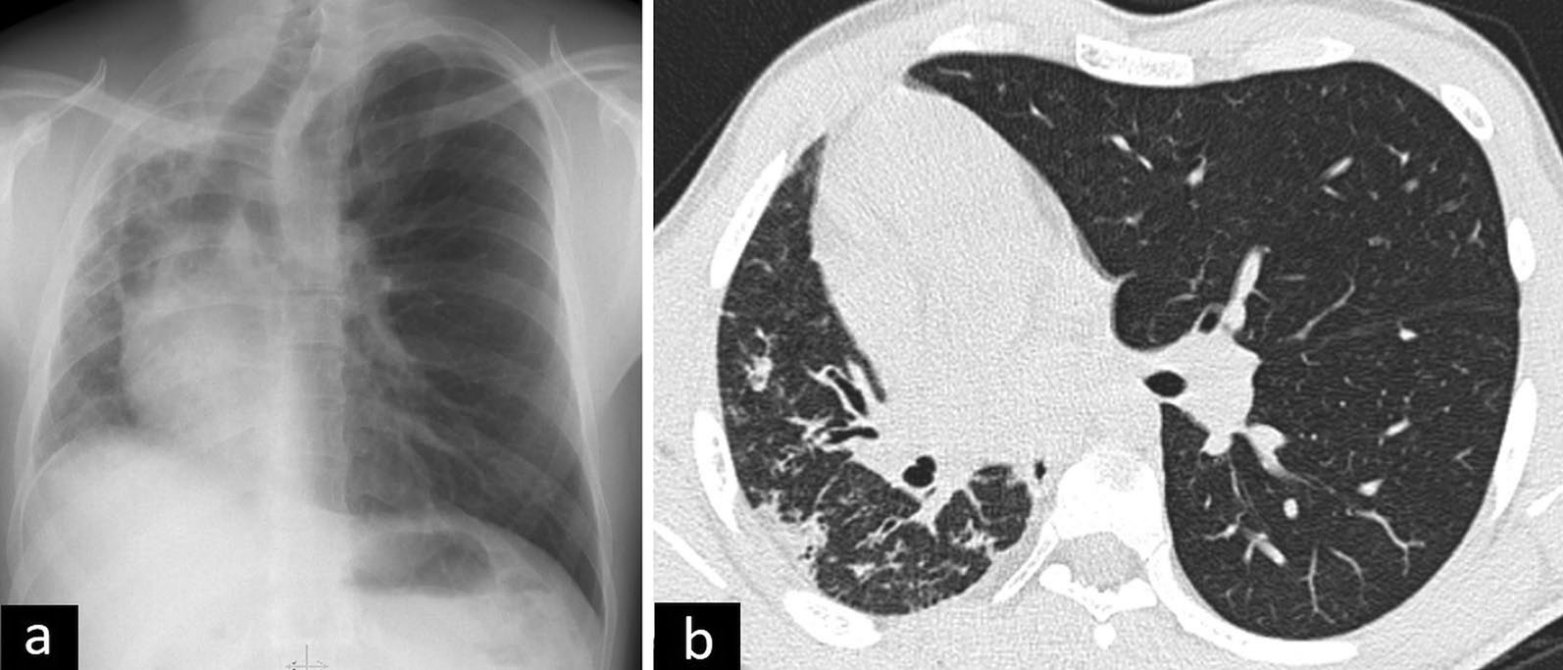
Imaging findings of pulmonary hypoplasia. Chest X-ray (a) and axial CT image (b) of a 26-year-old male presenting with recurrent infection demonstrate pulmonary hypoplasia of the right lung. Although bronchi and alveoli are present in underdeveloped lungs, the size of the right lung is decreased. Consolidations due to infection can also be seen on axial CT (b)
